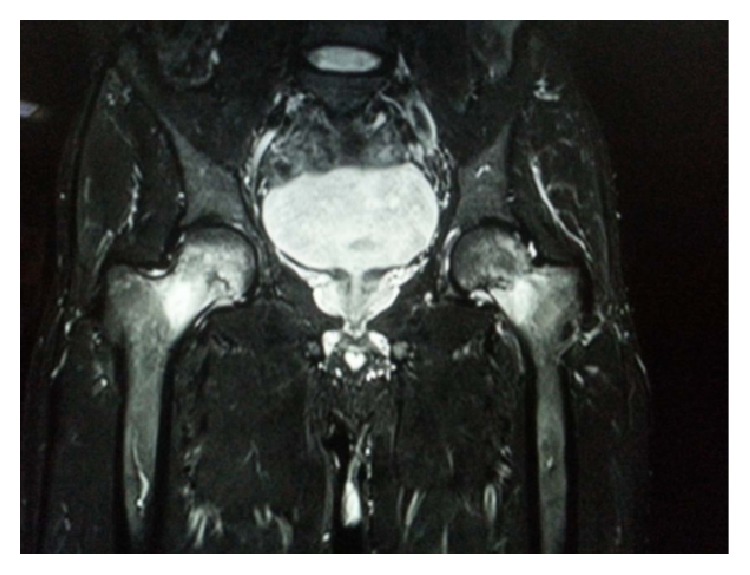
A T 2 weighted coronal magnetic resonance imaging (MRI) scan of pelvis with both hips showing oedema adjacent to the fracture line in the neck.
